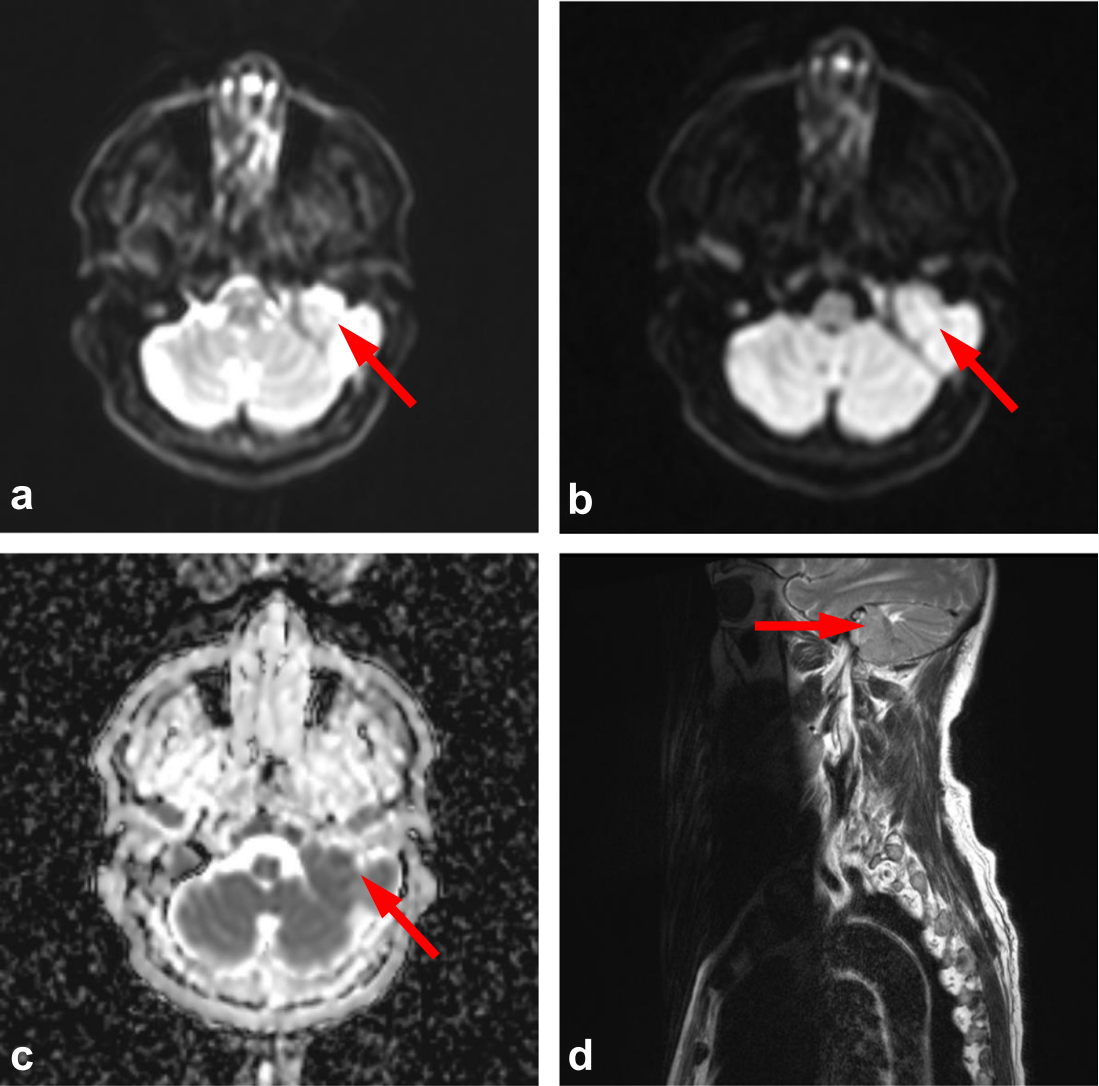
Base of skull lesion. Observer score variation within the skull due to large base of skull lesion (arrows) shown by (a) axial b50 DW MRI, b axial b900 DW MRI, c corresponding ADC map and (d) sagittal T2 weighted MRI of upper spine. Both brain and skull lesion have high diffusion signal (a-b) with low ADC (c), due to close proximity one observer reported images as normal
